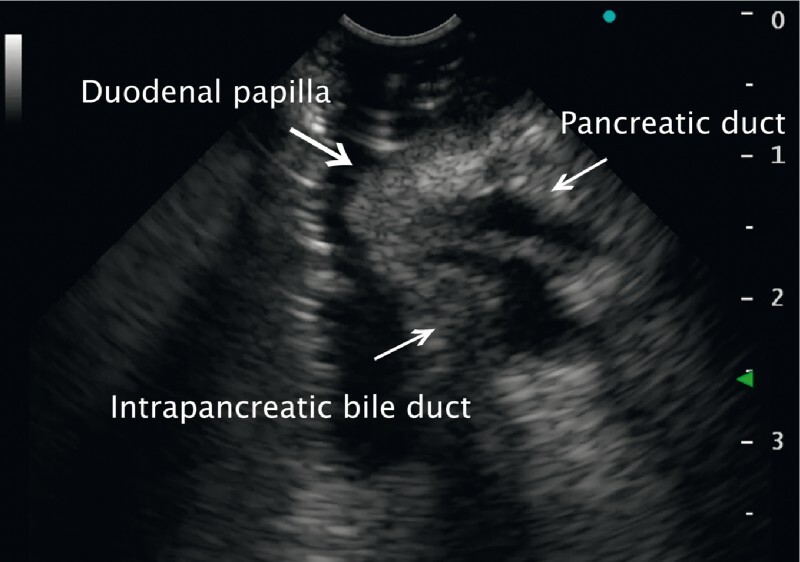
Gel immersion endoscopic ultrasound view clearly showing the papilla and intrapancreatic bile duct.
